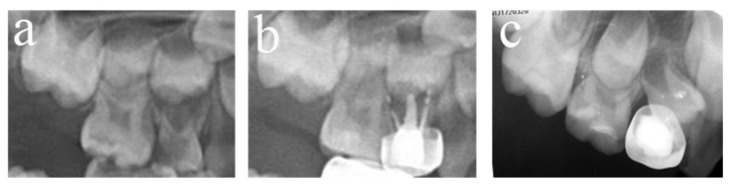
#54 received pulpectomies under DGA with a 34-month follow-up period (65-month-old male child). (a) The preoperative radiograph revealed periapical radiolucency surrounding #54. (b) Radiograph taken one week postoperatively showing overfilling in the buccal root canals. (c) Twenty-six months after the operation, the root was completely absorbed, and the eruption direction of #14 was altered. Pulpectomies in #54 failed.
